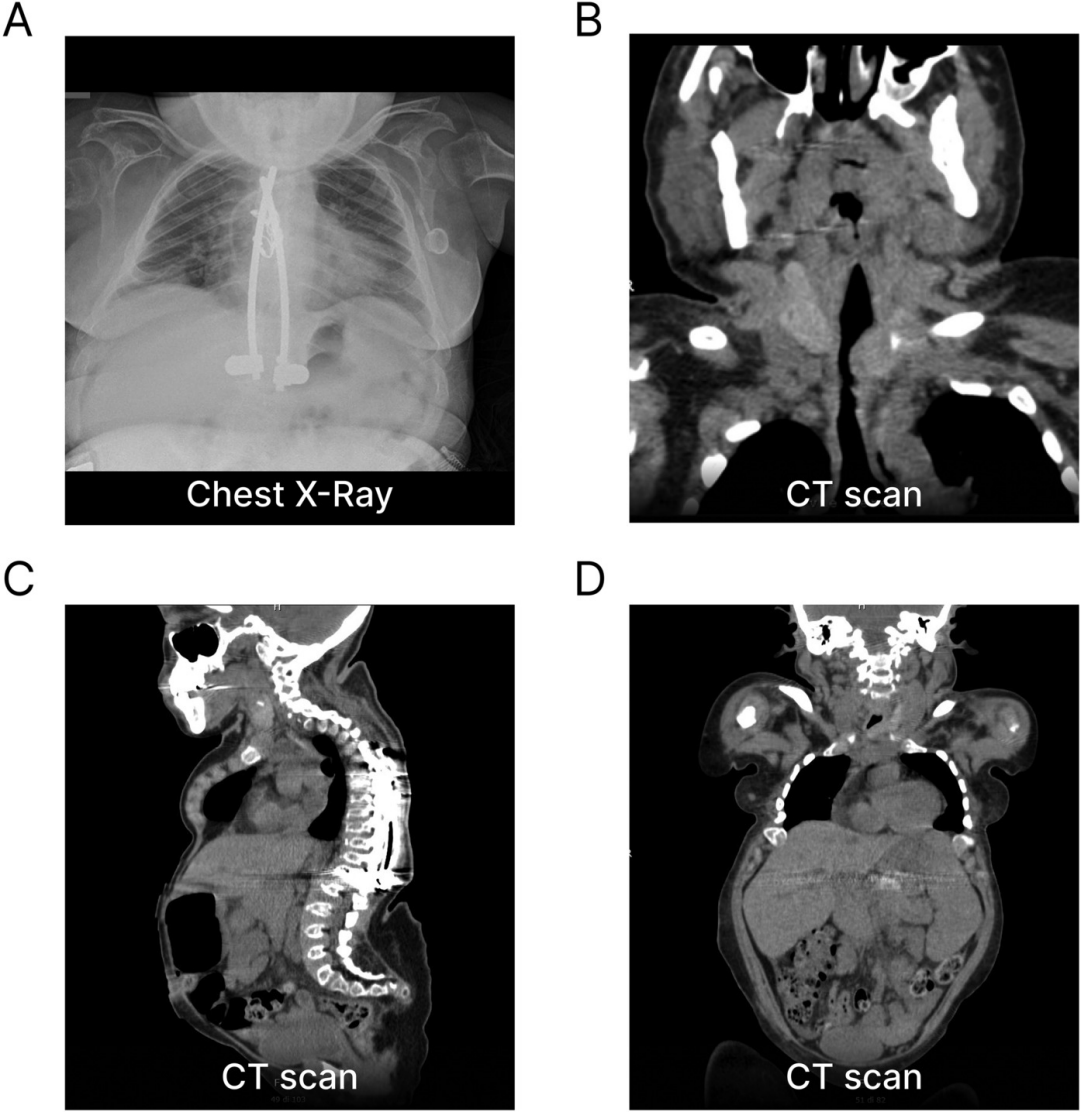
Imaging sections of phenotypic manifestations of our patient with Morquio syndrome. (A) Chest x-ray at B-ALL onset showing the absence of a mediastinal mass and evidence of spinal instrumentation with fixation from T9 to L1. (B) Frontal CT scan demonstrating a reduced-caliber trachea secondary to extra-luminal GAG accumulation. (C) Sagittal CT scan revealing skeletal dysplasia and severe anatomical abnormalities of the vertebral spine and thorax, including marked thoracic kyphoscoliosis and cervical and sacrolumbar hyperlordosis. (D) Total-body CT scan at B-ALL onset showing stable hepatosplenomegaly. GAG, glycosaminoglycans.
